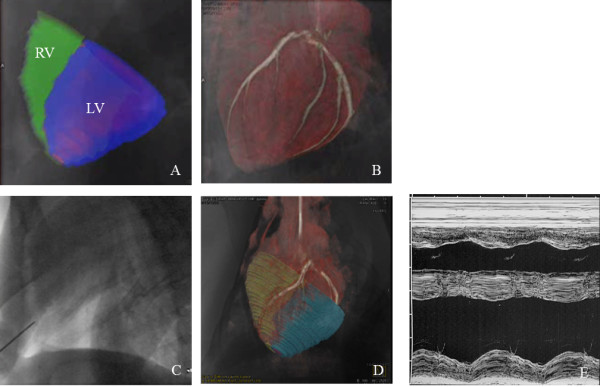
X-ray fused with MRI (XFM) of X-ray-visible microcapsules to the heart. (A) Segmented cine CMR showing epicardial contours (green-RV; blue-LV) overlaid on live X-ray fluoroscopic image. (B) Coronary vasculature from c-arm CT overlaid on live X-ray fluoroscopic image. (C) Live X-ray fluoroscopy demonstrating radiopacity of needle used for pericardial puncture and the lack of ability to visualize the myocardium or coronary vasculature without XFM. (D) Live X-ray fluoroscopy image overlaid on segmented whole heart CMR and c-arm CT volumes showing pericardial puncture. (E) An M-mode echocardiogram at seven days post-injection demonstrating normal cardiac function and no abnormalities to the pericardium. Reprinted with permission from Azene *et al.*[173].
